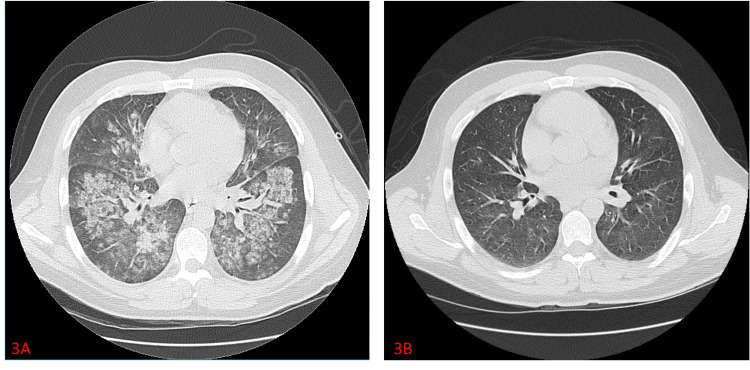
CT scan of the patient’s chest before (3A) and after corticosteroid treatment (3B), showing marked improvement from previous diffuse ground-glass opacities.
